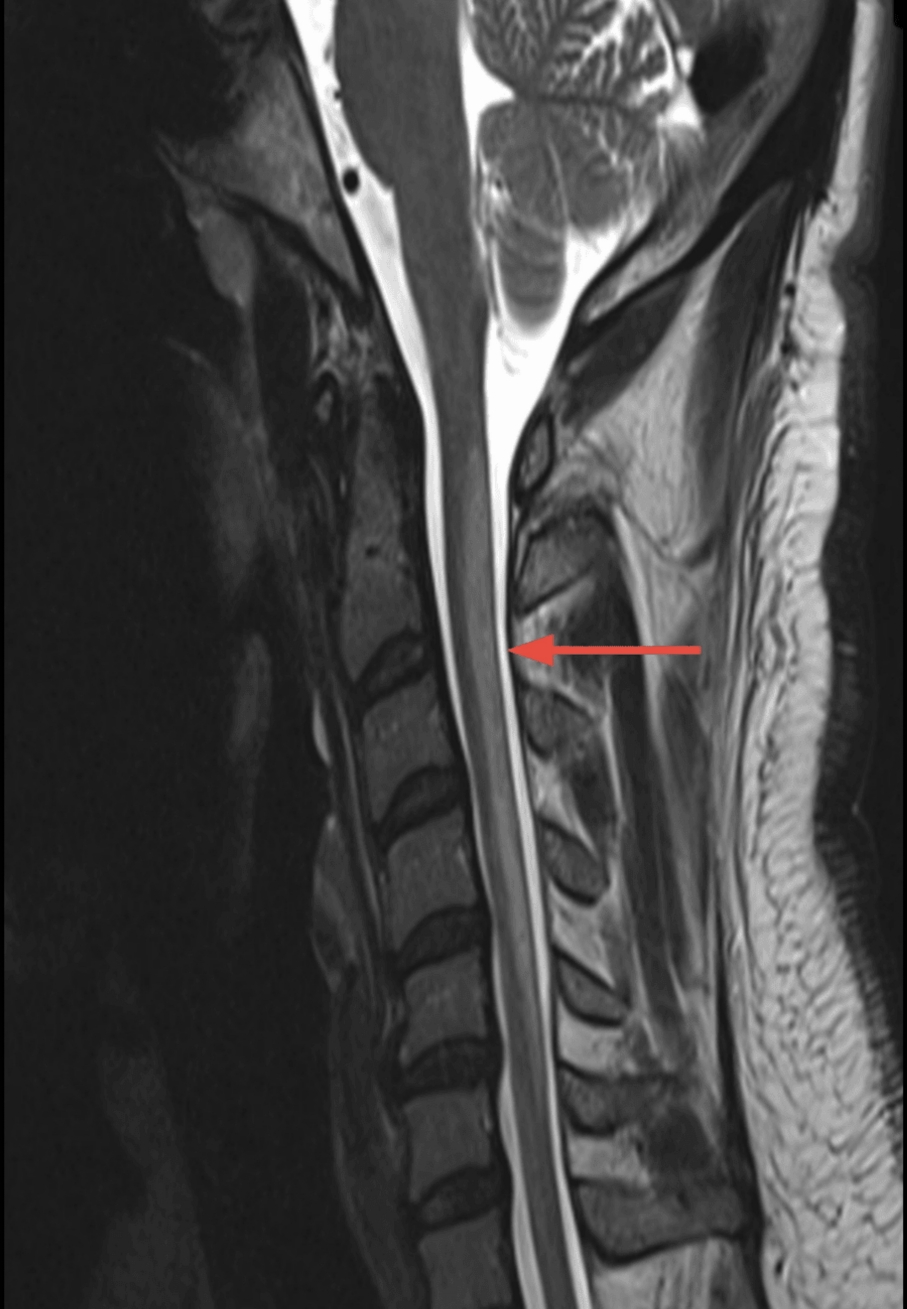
Sagittal T2-Weighted MRI of Cervical Spine Demonstrating Dorsal Column Hyperintensity Sagittal T2-weighted MRI of the cervical spine shows linear bilateral hyperintensity involving the dorsal columns from C2 to C5 (red arrow), without mass effect or contrast enhancement. These findings are characteristic of subacute combined degeneration due to vitamin B12 deficiency. No evidence of cord expansion or compressive lesion is noted.
